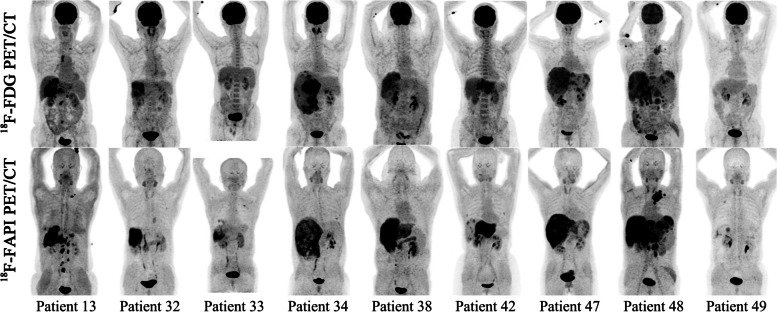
Nine representative patients with HCC underwent ^18^F-FDG & ^18^F-FAPI PET/CT imaging. ^18^F-FAPI PET/CT outperformed.^18^F-FDG PET/CT in detecting primary tumors (Patient No. 32, 33, 34, 49), intrahepatic subfoci (Patient No. 13, 38, 42, 47, 48), supraclavicular lymph node metastases (Patient No. 13, 48), retroperitoneum lymph node metastases (Patient No. 48), and comparable in detecting distant metastases (Patient No. 48)
